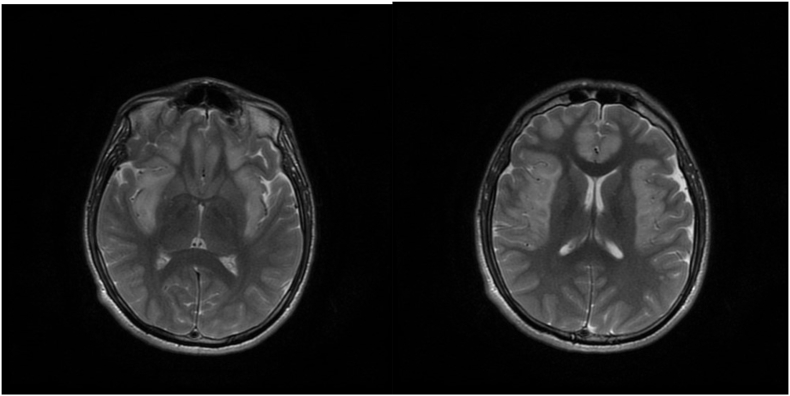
A, B: Fast recovery fast spin echo (FRFSE) MRI
